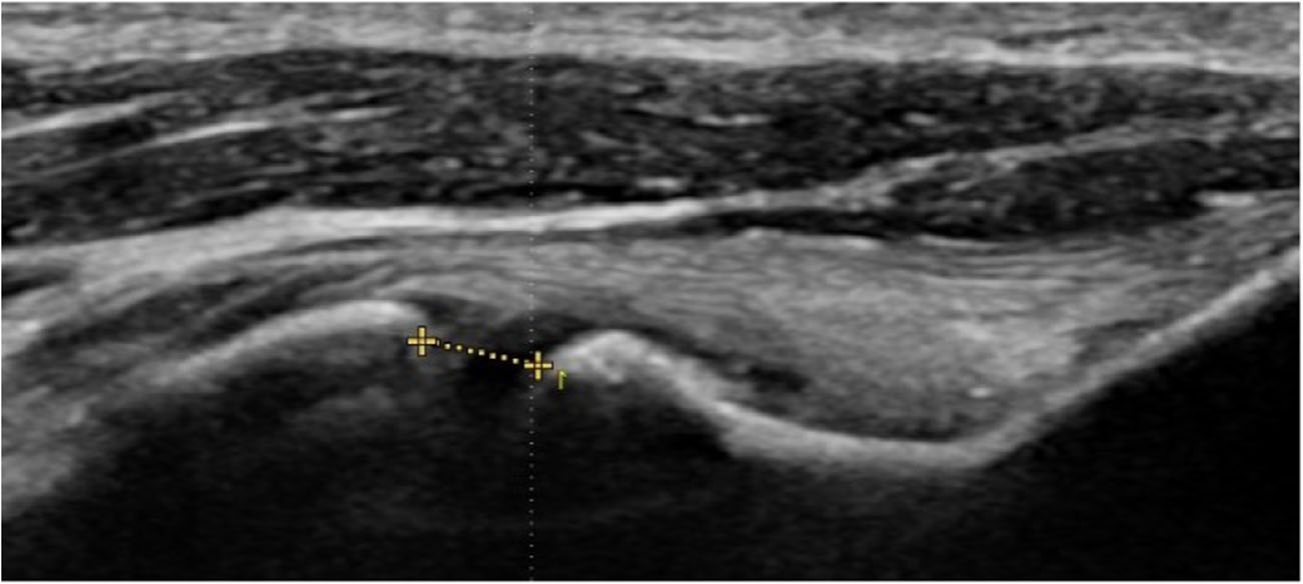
Ultrasound appearance of the mUCL with UHJ space noted with a dashed line. Right side is proximal
